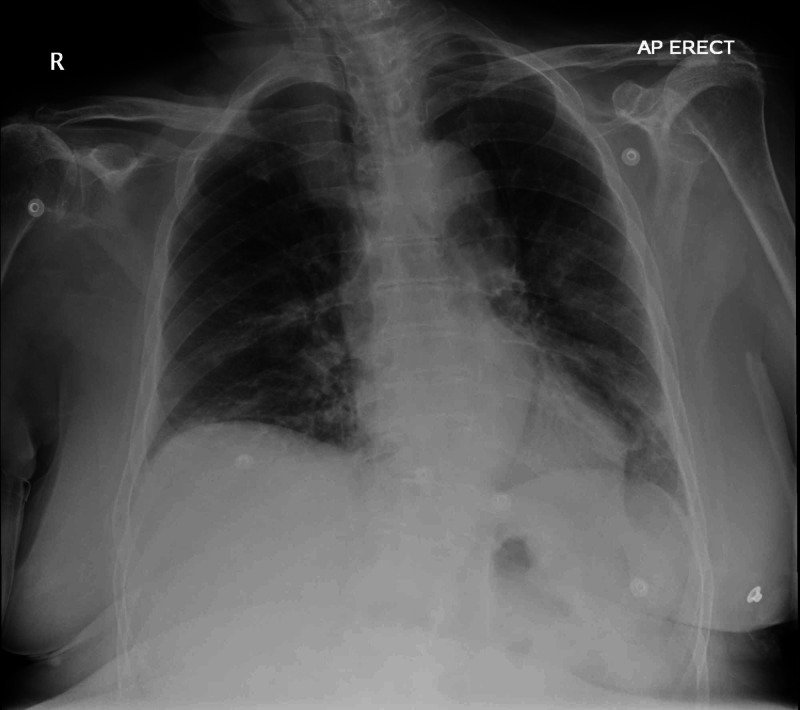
Chest X-ray. Rotated AP view. There are patchy peripheral airspace changes in the left middle, left lower and right lower zones, which are in keeping with COVID-19 infection.
